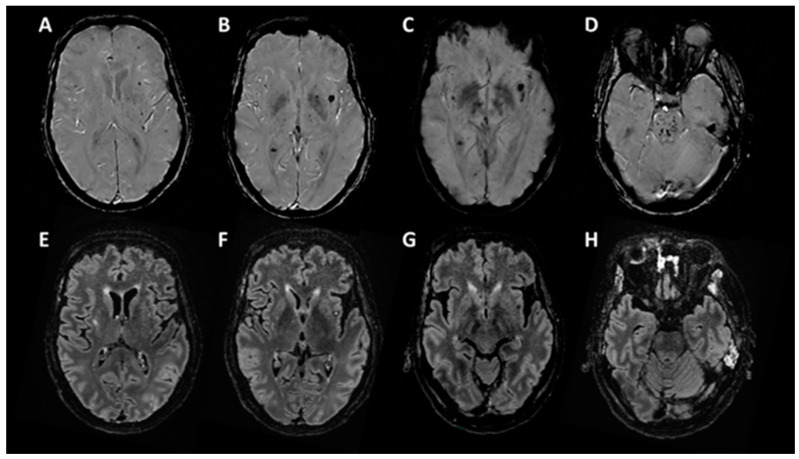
Brain MRI, T2 Star Susceptibility Weighted ANgiography (SWAN) sequence (A–D), and FLAIR sequence (E–H).
